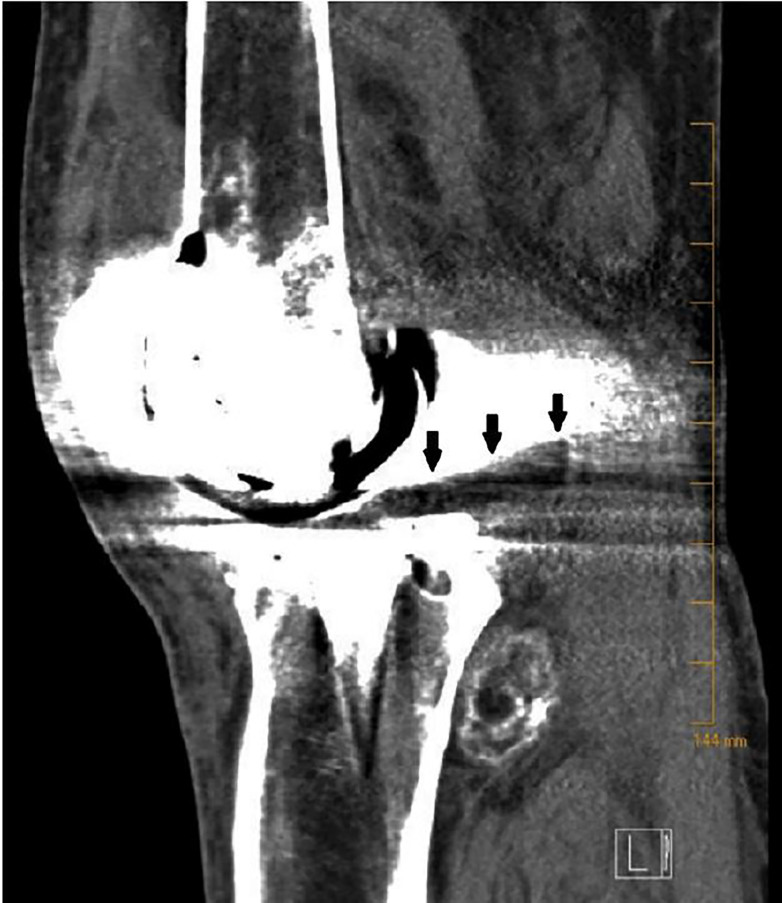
Computed tomography of left knee (sagittal view) showing posterior subluxation of the tibia with posterior displacement of the tibial polyethylene spacer (arrows).
